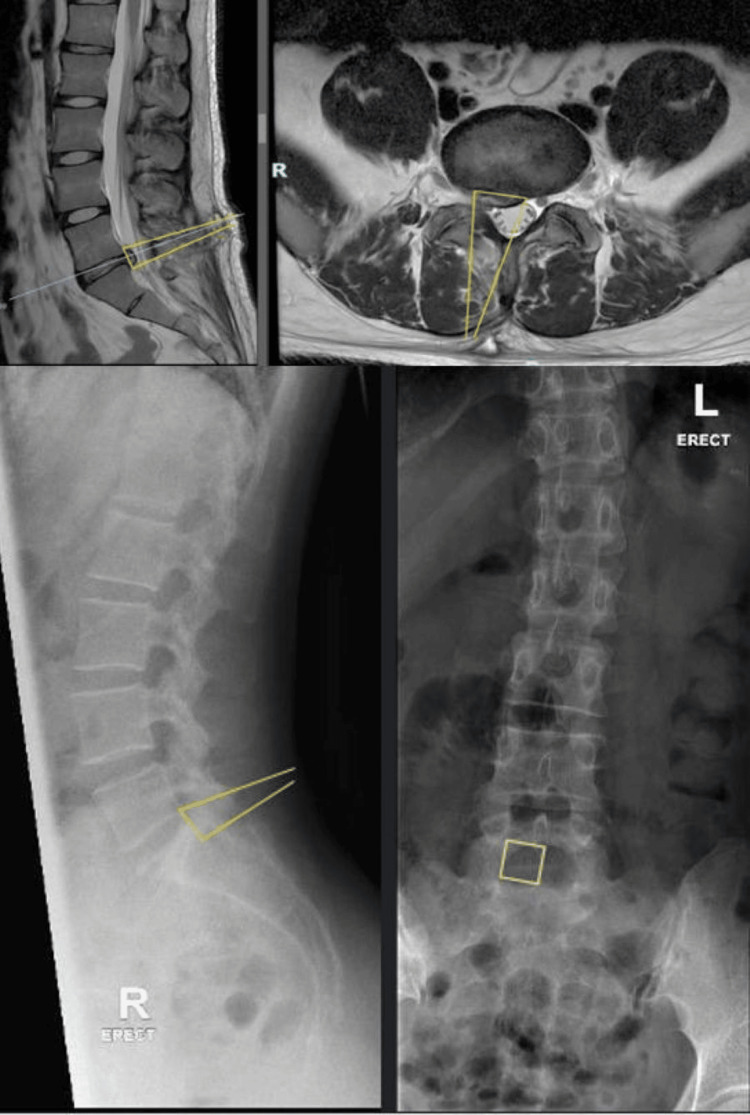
Pre-operative planning showcasing the approach and extent of decompression on MRI prior to a right L5-S1 revision endoscopic decompression and discectomy (top) in sagittal (left) and axial (right) cuts; superimposed on X-ray films (bottom) in lateral (left) and anterior-posterior (right) views MRI: magnetic resonance imaging
